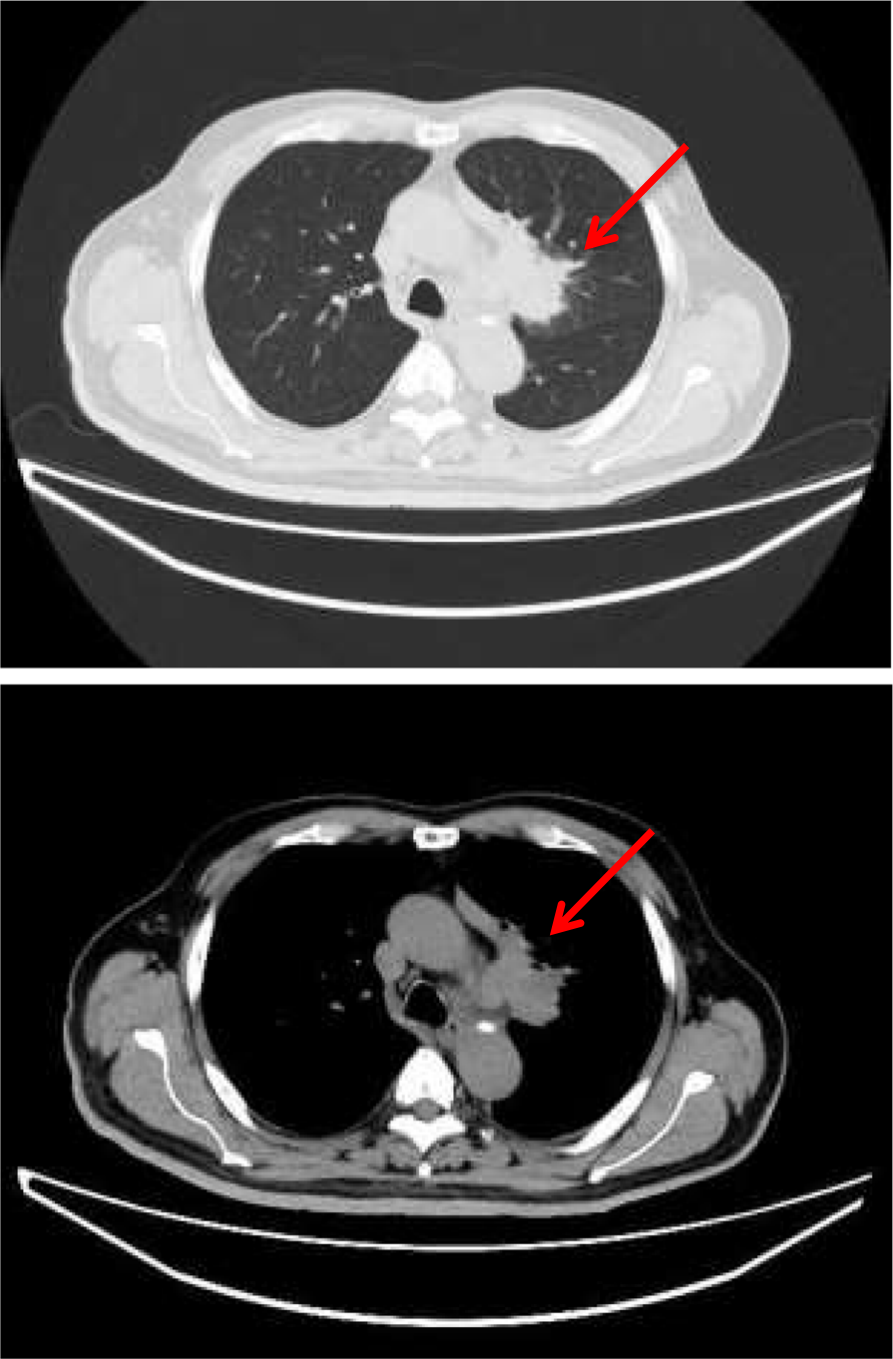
Chest computed tomographic scan revealing a mass in the left hilum (*red arrow*)
